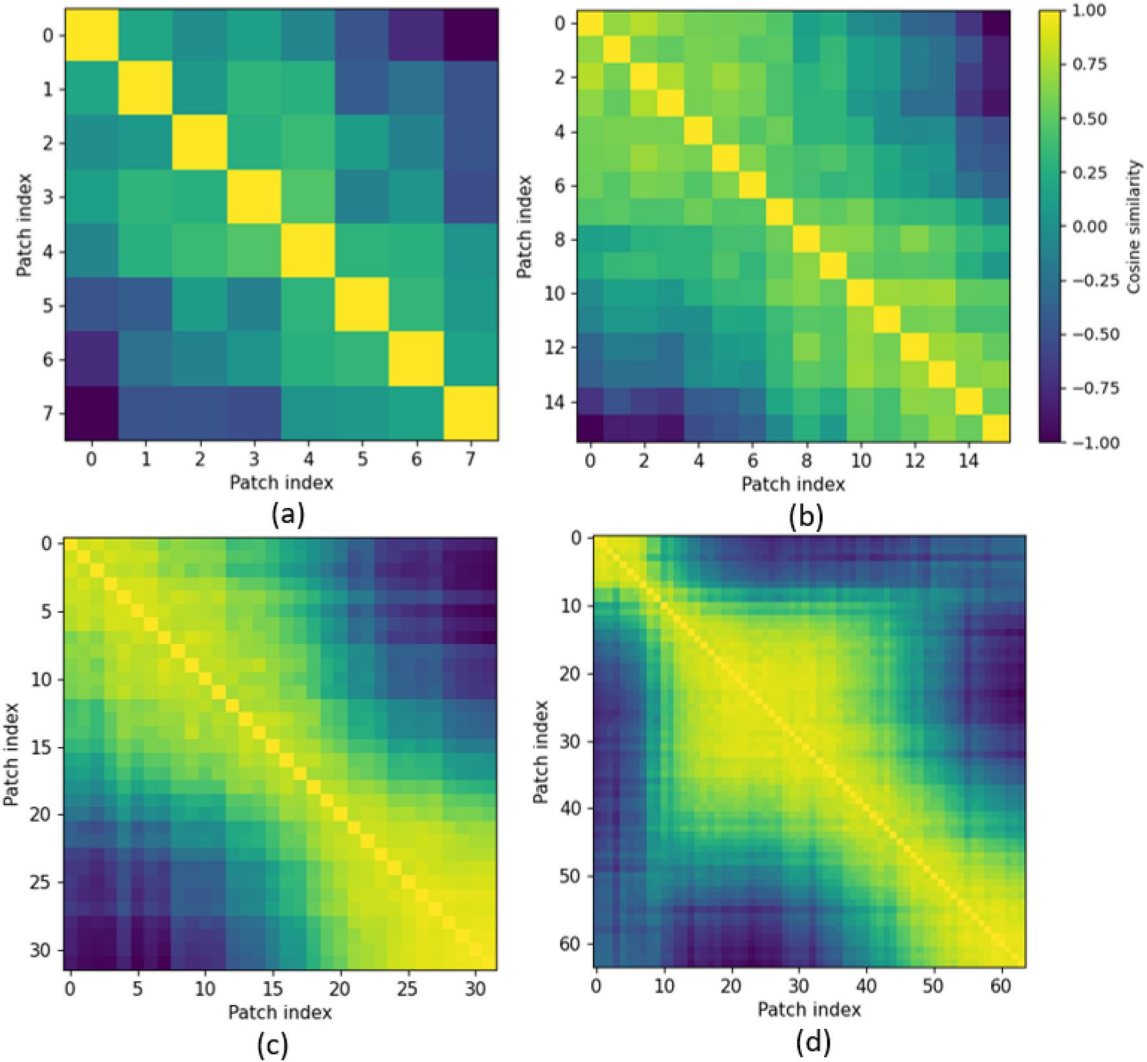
Cosine similarities of repetition 3, subject 20 of $$\text {CT-HGR}$$-V1 for (a) $$W=64$$ (b) $$W=128$$ (c) $$W=256$$ and (d) $$W=512$$.
